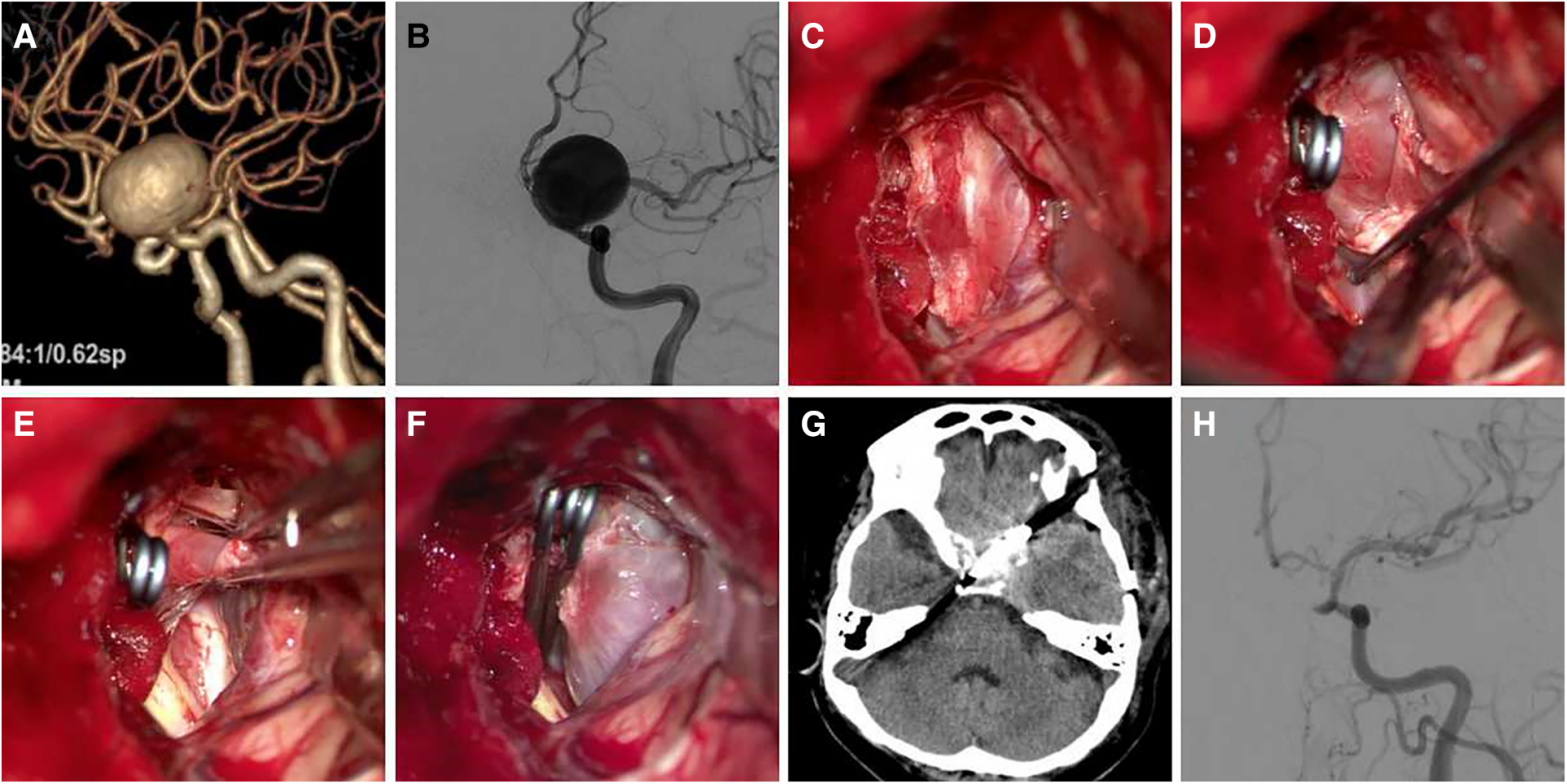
A 60-year-old female patient was diagnosed with giant C6 aneurysm. (A,B) Pre-operative CTA and DSA show a C6 aneurysm, the body point to dorsal-medial direction; (C) exposure aneurysm and clinoid segment of the ICA; (D) temporary occlusion of the clinoid segment of the ICA; (E,F) aneurysm neck is not wide, the aneurysm neck was completely clipped using two clips; (G,H) postoperative CT and DSA follow-up.
